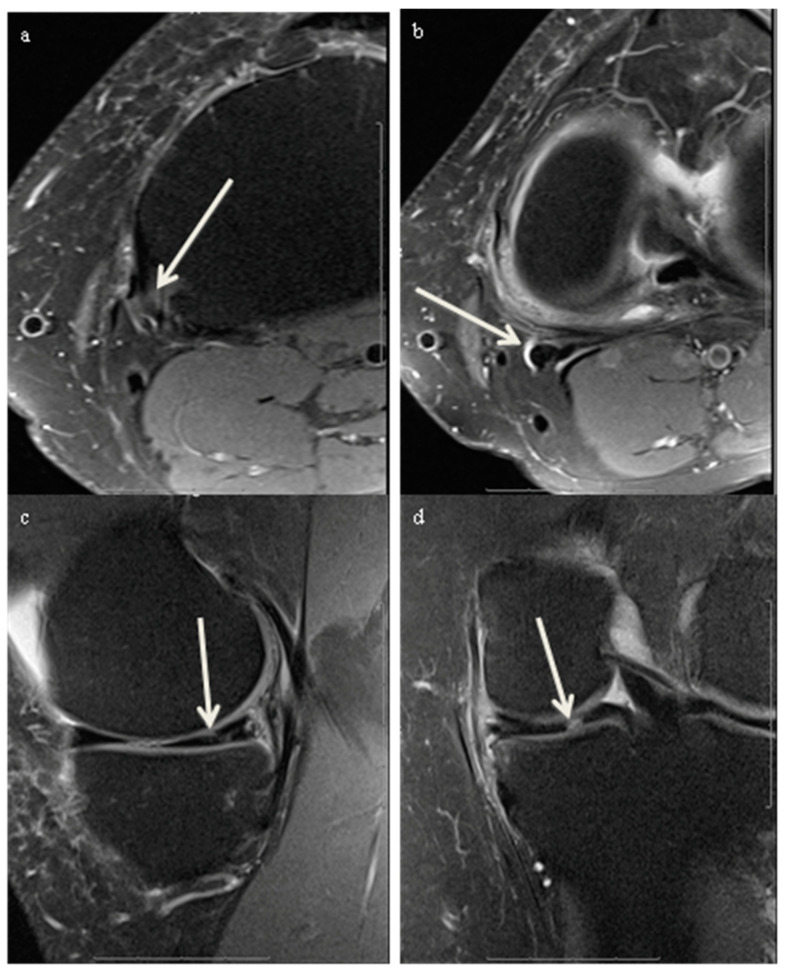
MRI scan of the left knee of a 34-year-old male patient who presented with medial sided knee pain and swelling. (a) Axial Proton Density Fat-Saturated MRI image, which demonstrated semimembranosus tendinosis (white arrow). (b) Axial Proton Density Fat Saturated image superior to (a) demonstrating presence of fluid within the semimembranosus bursa in keeping with bursitis (white arrow). (c,d) Sagittal and coronal Proton Density Fat Saturated MRI images, respectively, which demonstrate complex medial meniscal tear with horizontal and radial components involving the body and posterior horn (white arrow).
